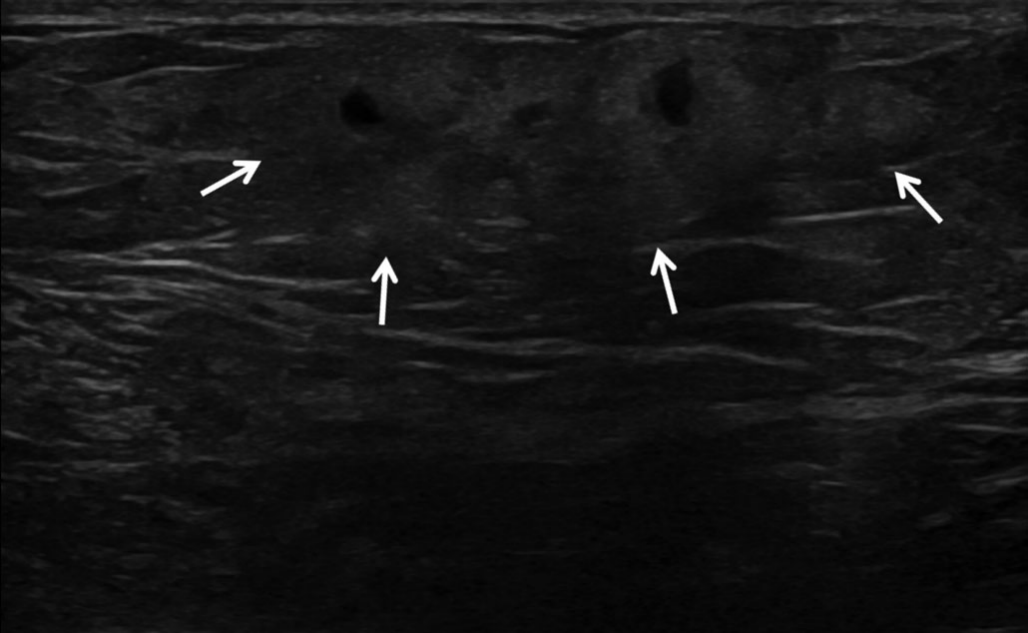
89‐year‐old woman with trauma to the left breast after a fall, which resulted in extensive bruising and multiple palpable lumps. Targeted ultrasound was performed with a ML6‐15 MHz transducer with the frequency set to 15 MHz and showed a 3 cm area of superficial hyperechogenicity (arrows) containing a few small cystic spaces, most consistent with acute fat necrosis.
